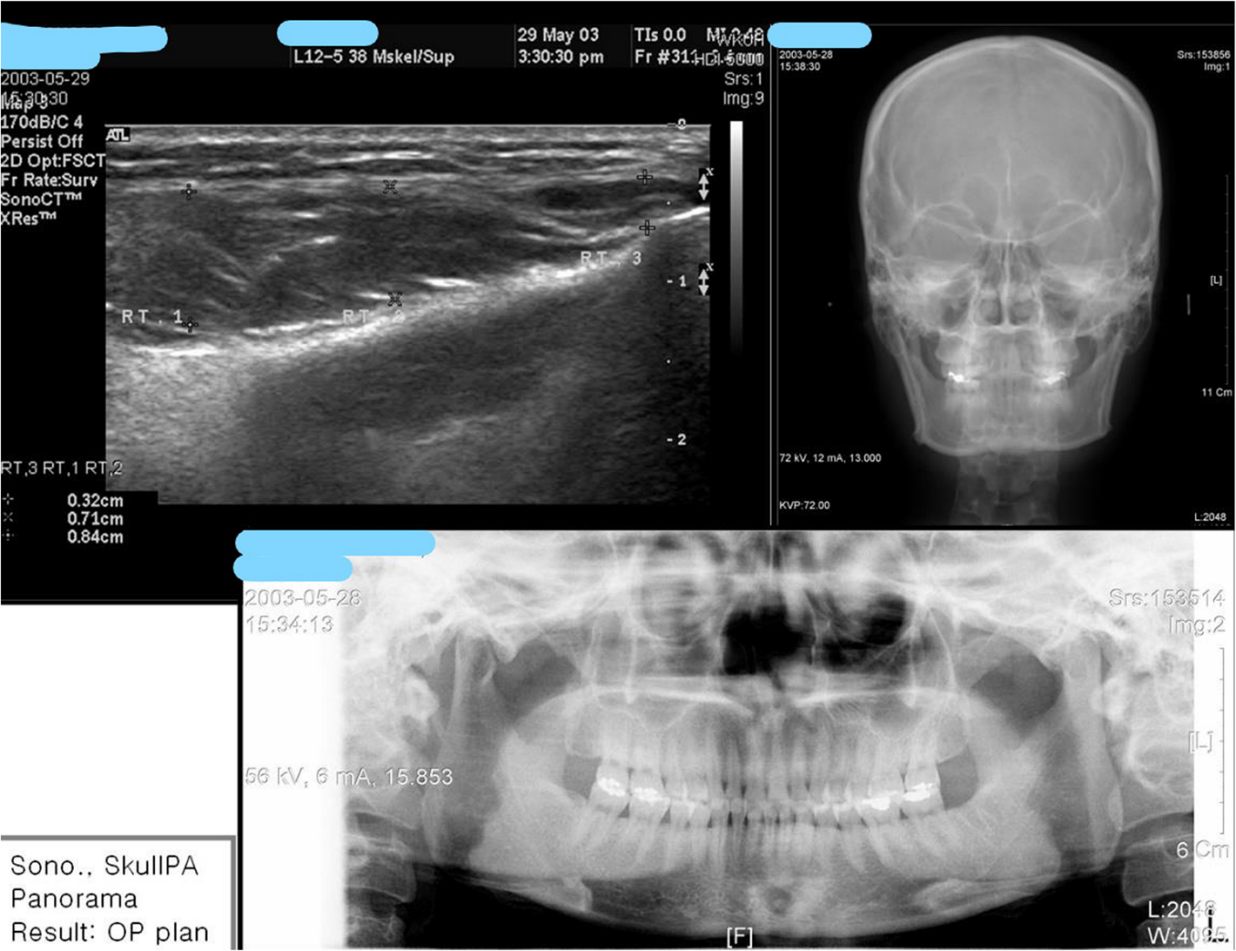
For an initial assessment of bruxism and square jaw, with tests such as sonography, skull PA, and panorama as seen above, one can examine the degree of contraction or hypertrophy of masseteric muscle of a patient with bruxism or square jaw, and able to identify the temporomandibular joint disorder, determine occlusal relationships, and diagnose pathological diseases. An average of 10 mm or more is determined as masseteric hypertrophy
